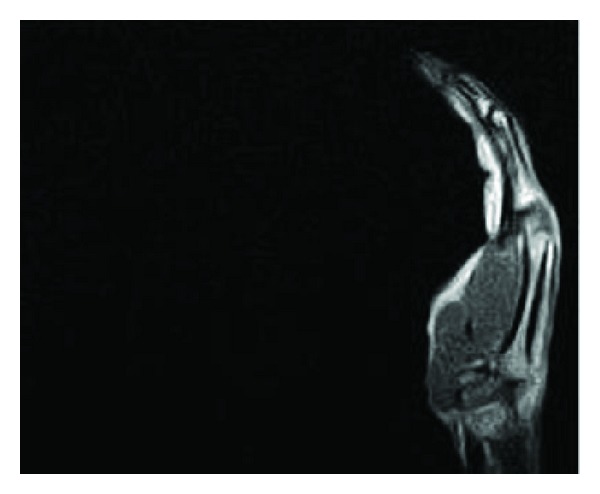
MR image of the volunteer without RFID tag attached to the wrist (imaging sequence: T_2_—Fast spin echo Sagittal).
